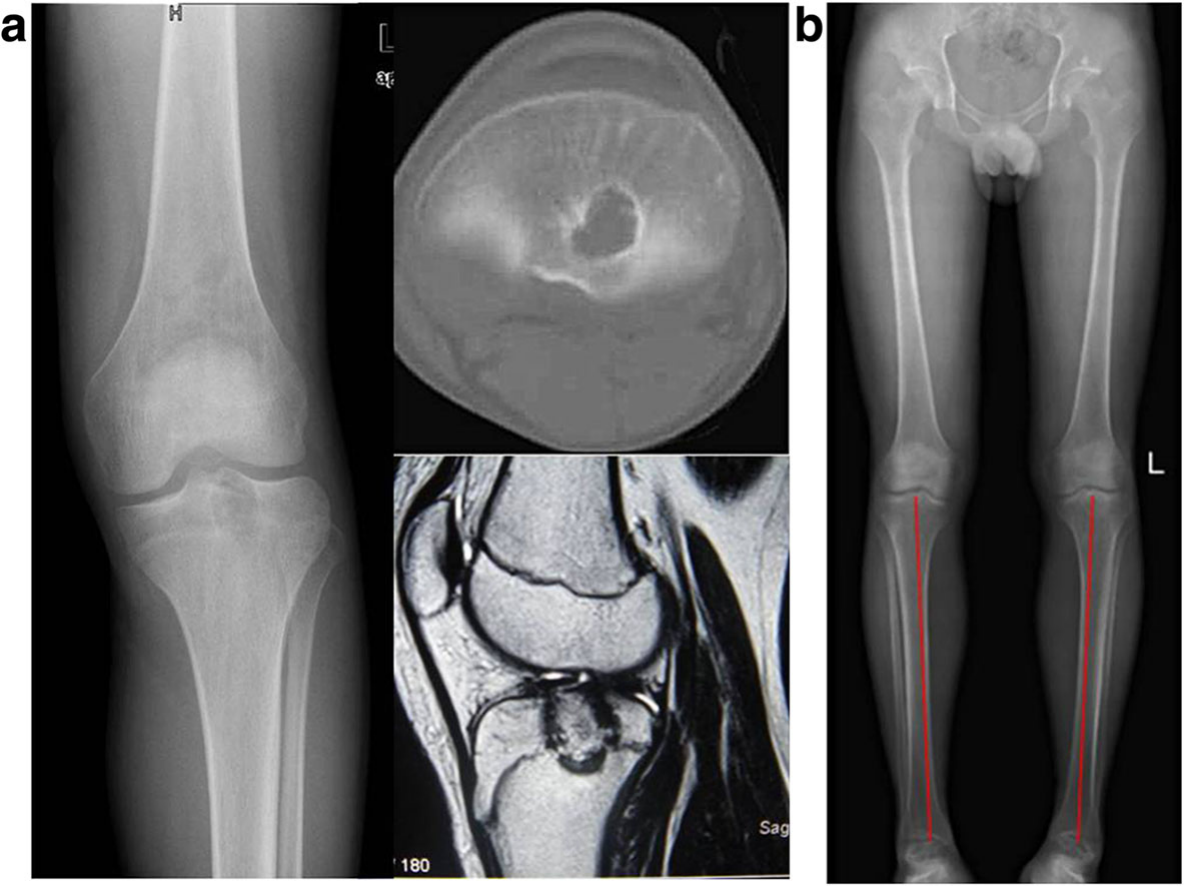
A 13-year-old boy’s X-ray, CT, and MRI (a) showed the lesion located at the proximal tibia. X-ray at 5.5 years postoperative told us that the grafting bone had been incorporated with the host bone and that the troubled limb shorten by 1.5 mm (b) compared with the healthy limb
